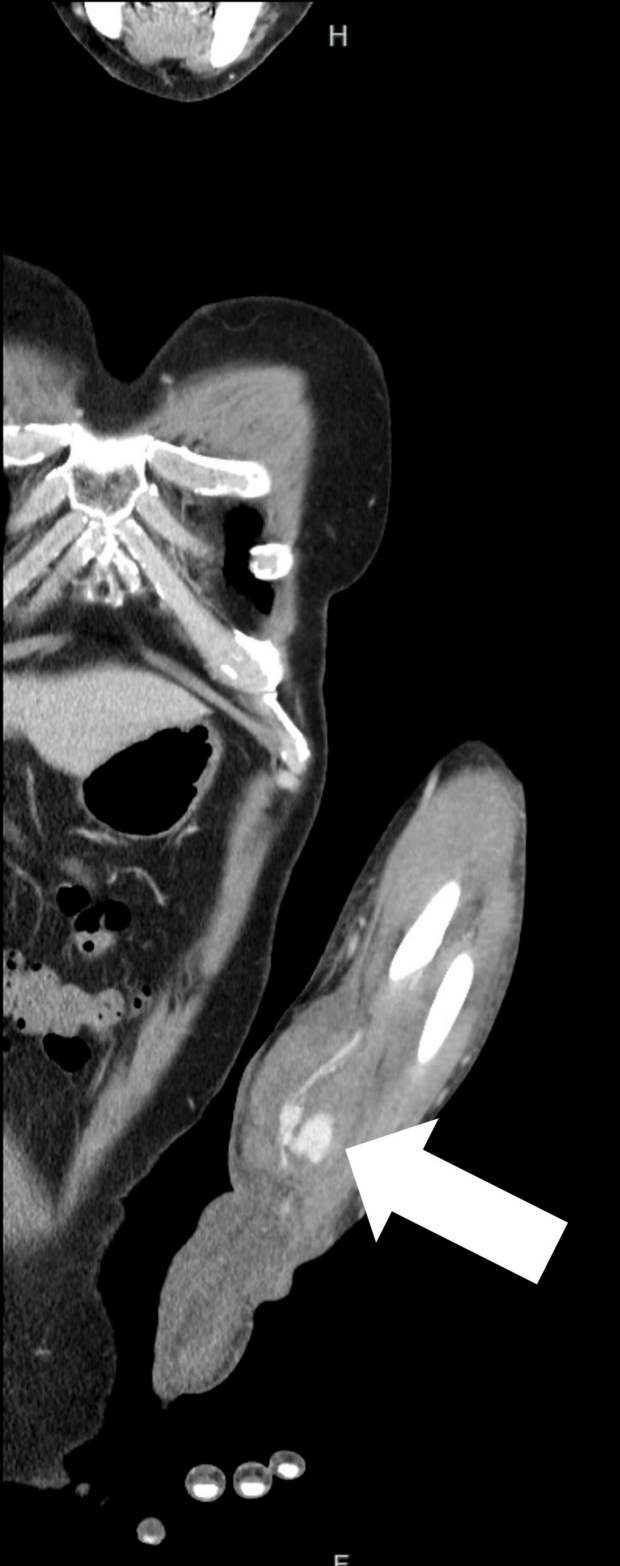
Contrast-enhanced computed tomography of the left forearm: a 29-mm pseudoaneurysm was seen that was continuous with the radial artery (white arrow).
